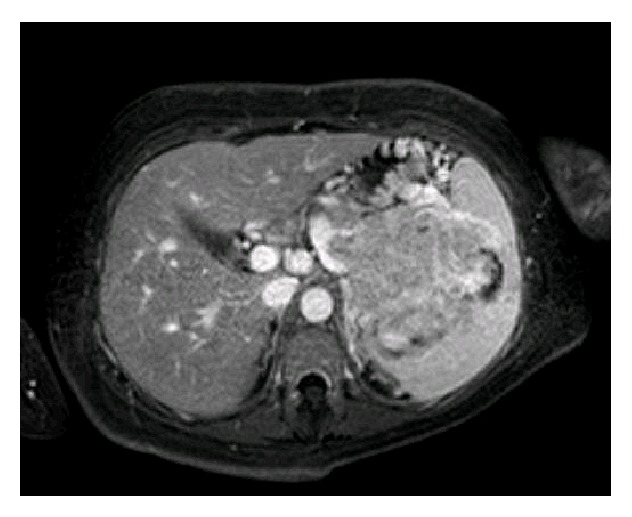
Magnetic resonance imaging of the abdomen was performed which revealed a 9 × 7 × 8 cm mass infiltrating the pancreatic tail and spleen along with massive upper abdominal varix formation secondary to splenic vein invasion.
